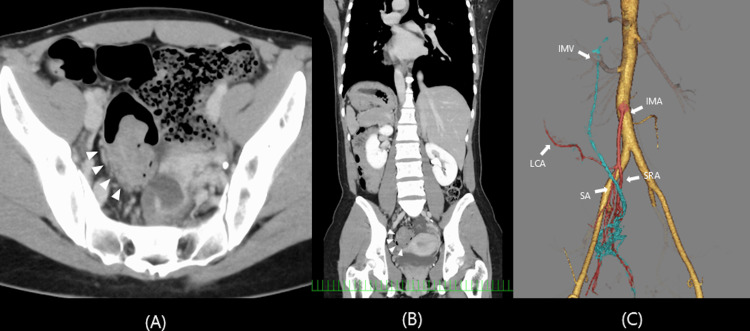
Contrast-enhanced computed tomography and 3D computed tomography angiography. Contrast-enhanced computed tomography showing a tumor in the distal sigmoid colon (arrowhead) on the axial image (A). Complete transposition of the thoracic and abdominal organs is demonstrated on the coronal image (B).  3D computed tomography angiography showed that the left colic artery and sigmoid artery had a common origin from the inferior mesenteric artery (C). IMA, inferior mesenteric artery; IMV, inferior mesenteric vein; LCA, left colic artery; SA, sigmoid artery; SRA, superior rectal artery; 3D, three-dimensional
